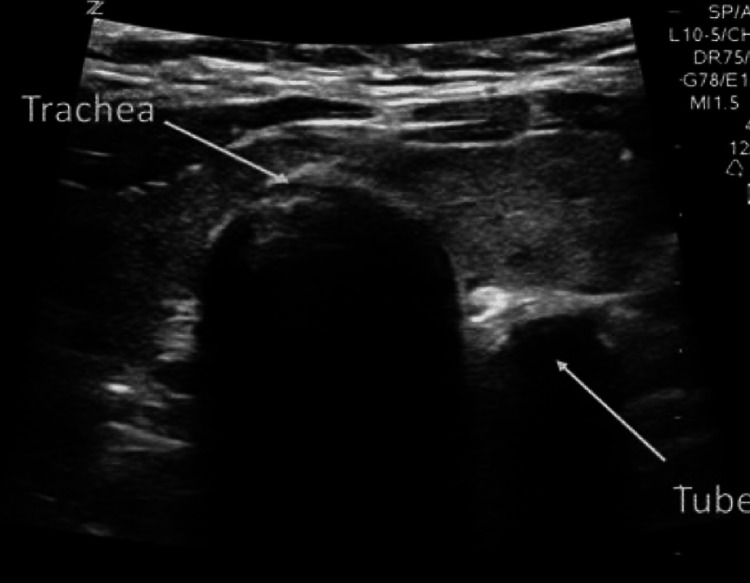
Ultrasonographic image of esophageal intubation
